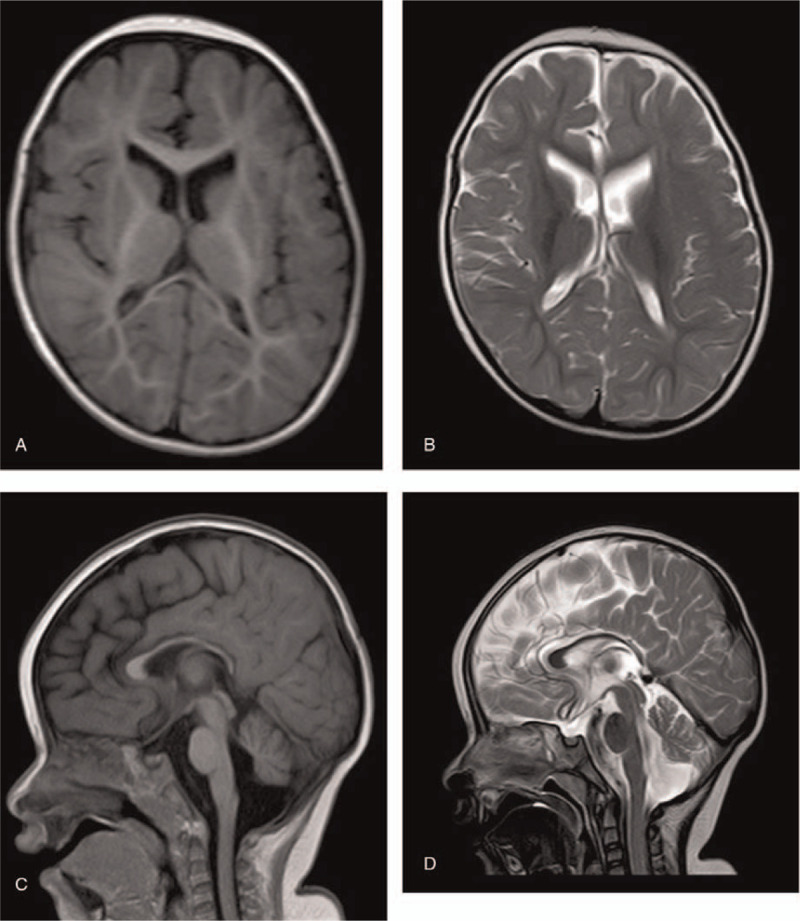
Magnetic resonance imaging of the patient's brain at 17 months A-D, which shows a sylvian fissure that is wider and deeper than normal, delayed myelinization, mild hypoplasia of the corpus callosum, and cerebellar hypoplasia.
